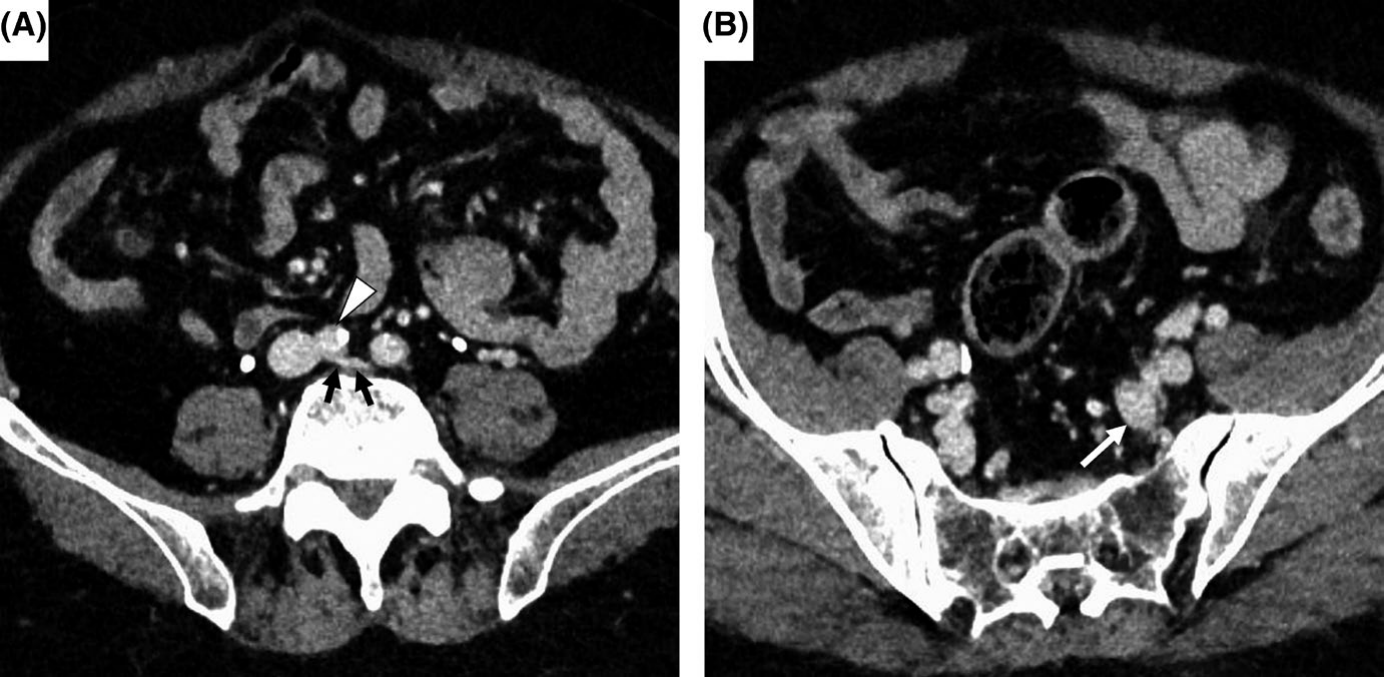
Findings of abdominal computed tomography with contrast enhancement. Computed tomography with contrast enhancement revealed that the left common iliac vein was compressed (A, black arrows) by the right common iliac artery (A, white arrowhead) and the lumbar vertebral body. The distal part of the left internal iliac vein was dilated (B, white arrow)
